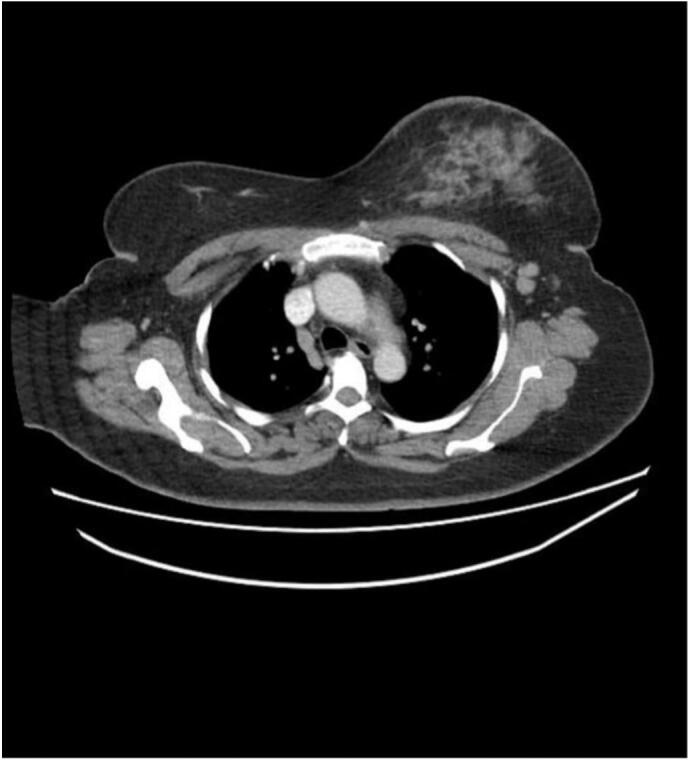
CT scan: interval development of asymmetric enhancement in the upper half of the right breast measuring 34 × 22 mm. Interval increase in the left axillary lymphadenopathy, the largest node measuring 12 mm in short axis.
